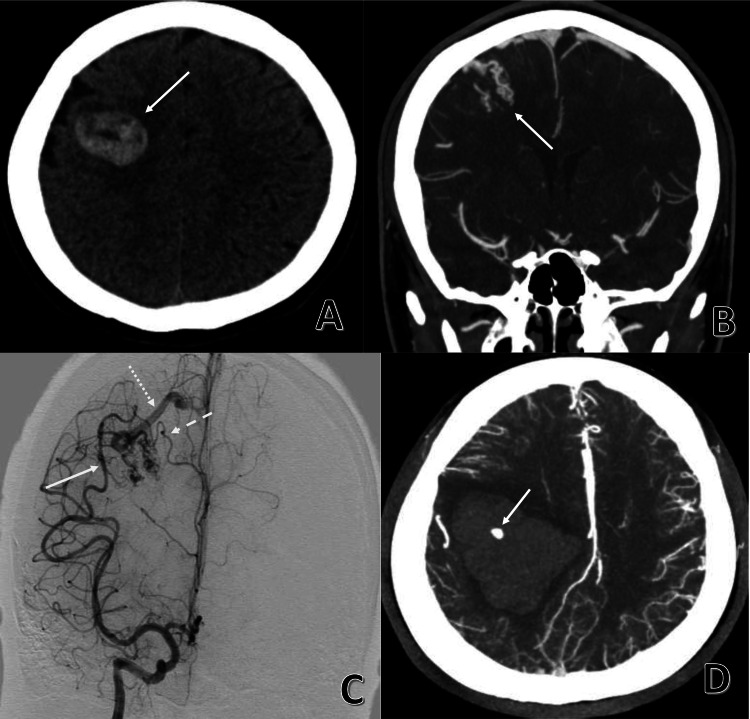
Computed tomography (A, B and D) and catheter angiogram (C) demonstrating the Spetzler-Martin grade 1 brain arteriovenous malformation and the associated intraparenchymal haematoma (A) Computed tomography showing a right frontal haematoma (solid arrow). (B) Computed tomography angiogram revealing a right frontal brain arteriovenous malformation (solid arrow). (C) Digital subtraction angiogram demonstrating the brain arteriovenous malformation fed by branches of the middle cerebral artery (solid arrow) and anterior cerebral artery (dashed arrow) with superficial drainage into the superior sagittal sinus (dotted arrow). (D) Urgent computed tomography after clinical deterioration showing haematoma expansion, intra-ventricular extension and evidence of contrast extravasation suggestive of active bleeding (solid arrow).
